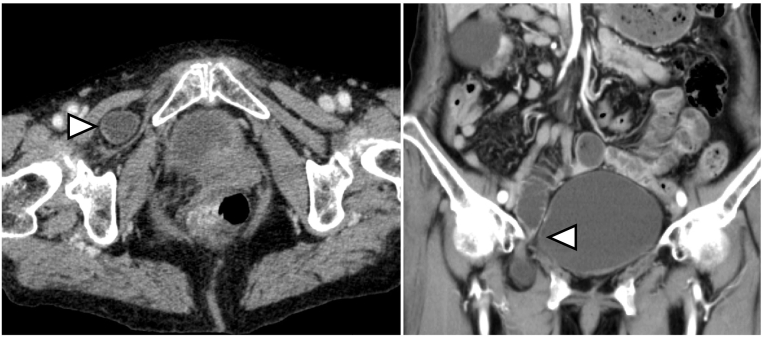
Computed tomography detected right obturator hernia. The oral side of strangulated intestine was slightly dilated.
